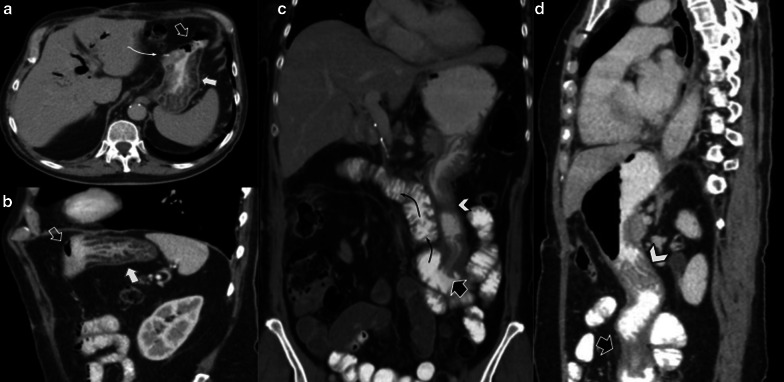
Gastrojejunostomy and duodenojejunostomy: (a, b) Gastrojejunostomy. Axial and sagittal portal venous phase CT scan, respectively. (c, d) Duodenojejunostomy. Coronal and oblique sagittal view portal venous phase CT scan, respectively. Note the importance of using positive oral contrast to denote marked mucosal pattern change in A and B, and to depict the pylorus and latero-terminal anastomosis in duodenojejunostomy. References: White curved arrow: Surgical clips; Thick white arrows: gastric remnant; black arrows: ascended jejunal loop; Thick black arrow: latero-terminal anastomosis; arrowhead: pylorus; curved black arrows: oral contrast reflux to afferent limb. Black arrows: ascended jejunal loop
